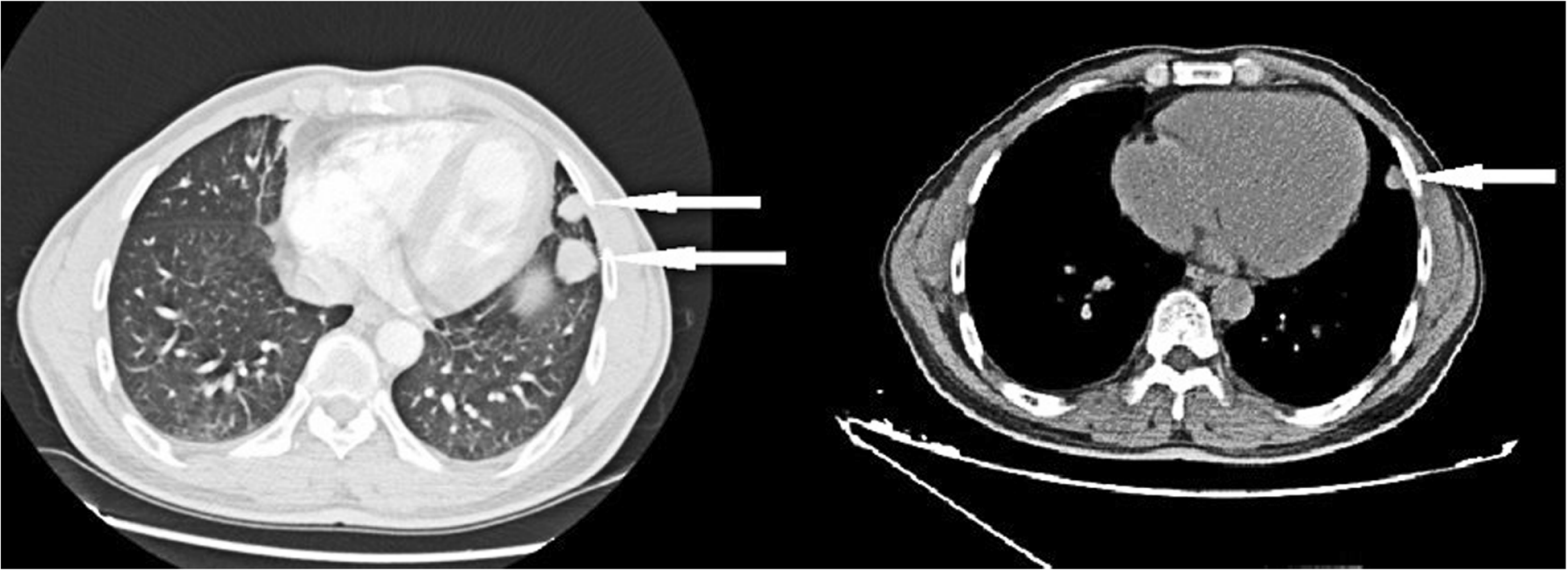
computed tomography scan (white arrow) of the chest showing the occupancy of mediastinum and the lungs
